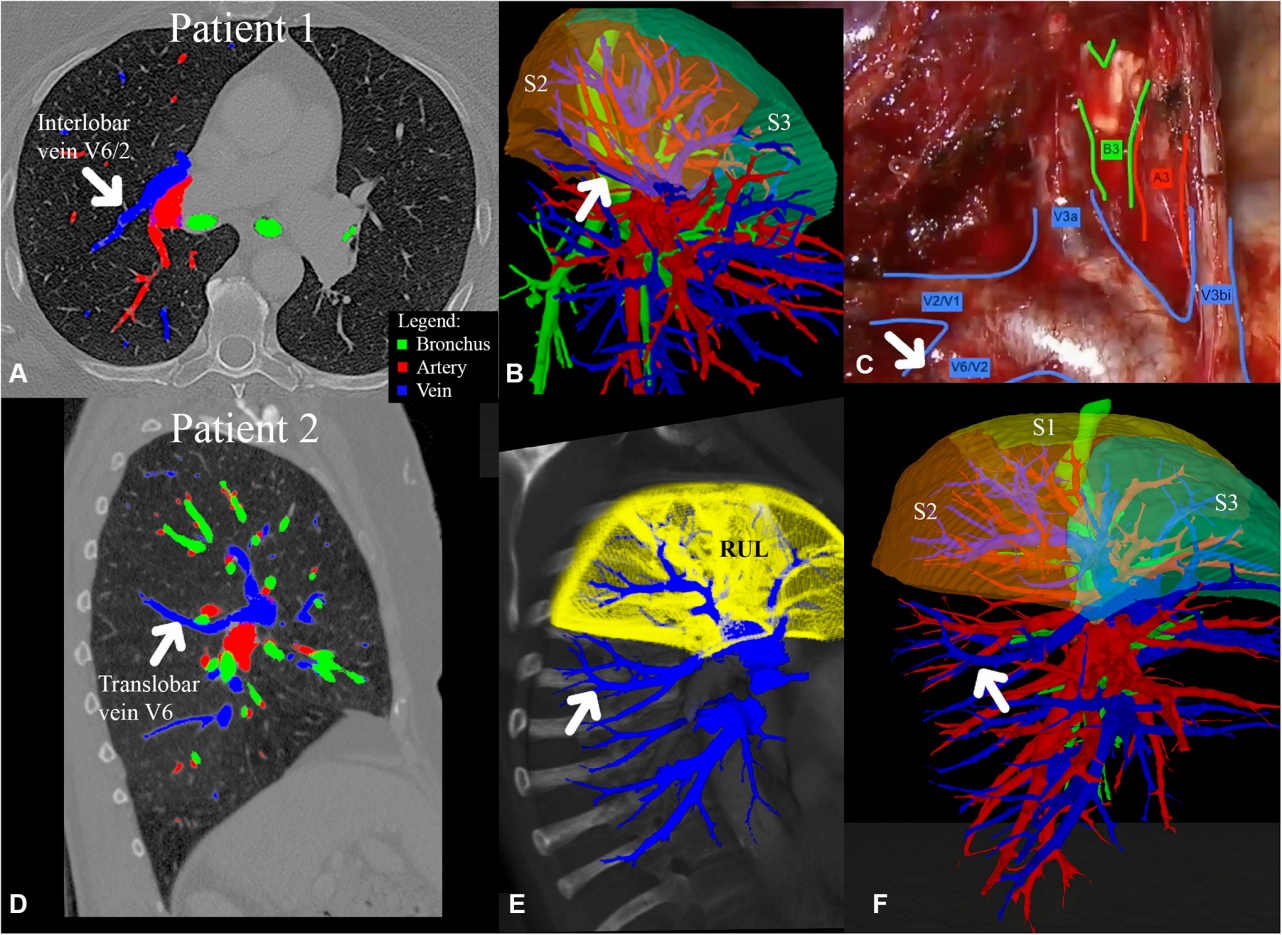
Two-dimensional computed tomography (2D-Ct), virtual reality, and 3-dimensional visualization of cases 1 and 2. A, Case 1: 2D-CT. B, Pulmo3D, lateral view. C, Intraoperative representation of interlobar vein V6/2 (*white arrow* points to interlobar vein V6/2). D, Case 2: 2D-CT. E, PulmoVR lateral view. F, Pulmo3D, lateral view of translobar vein of S6 (*white arrow* points to translobar vein V6). *A*, Artery; *B*, bronchus; *V*, vein; *RUL*, right upper lobe; *S*, segment.
